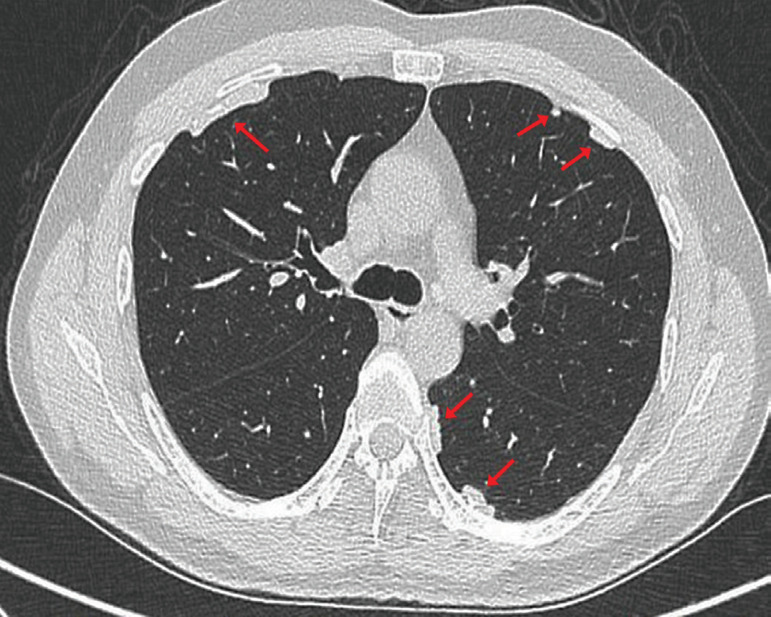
Axial section of chest computed tomography showing several calcified pleural plaques in different territories of the same patient.
